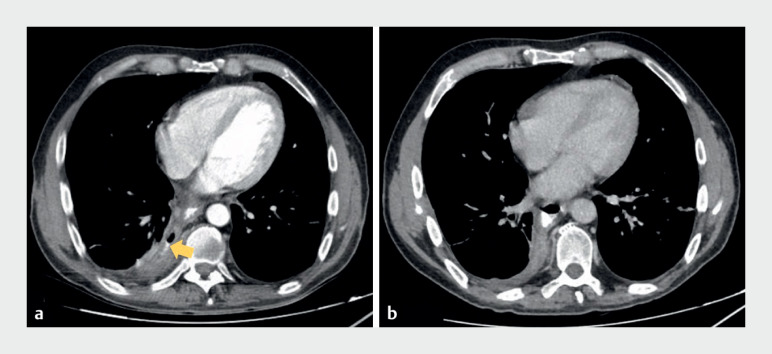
Computed tomography (CT) images.
